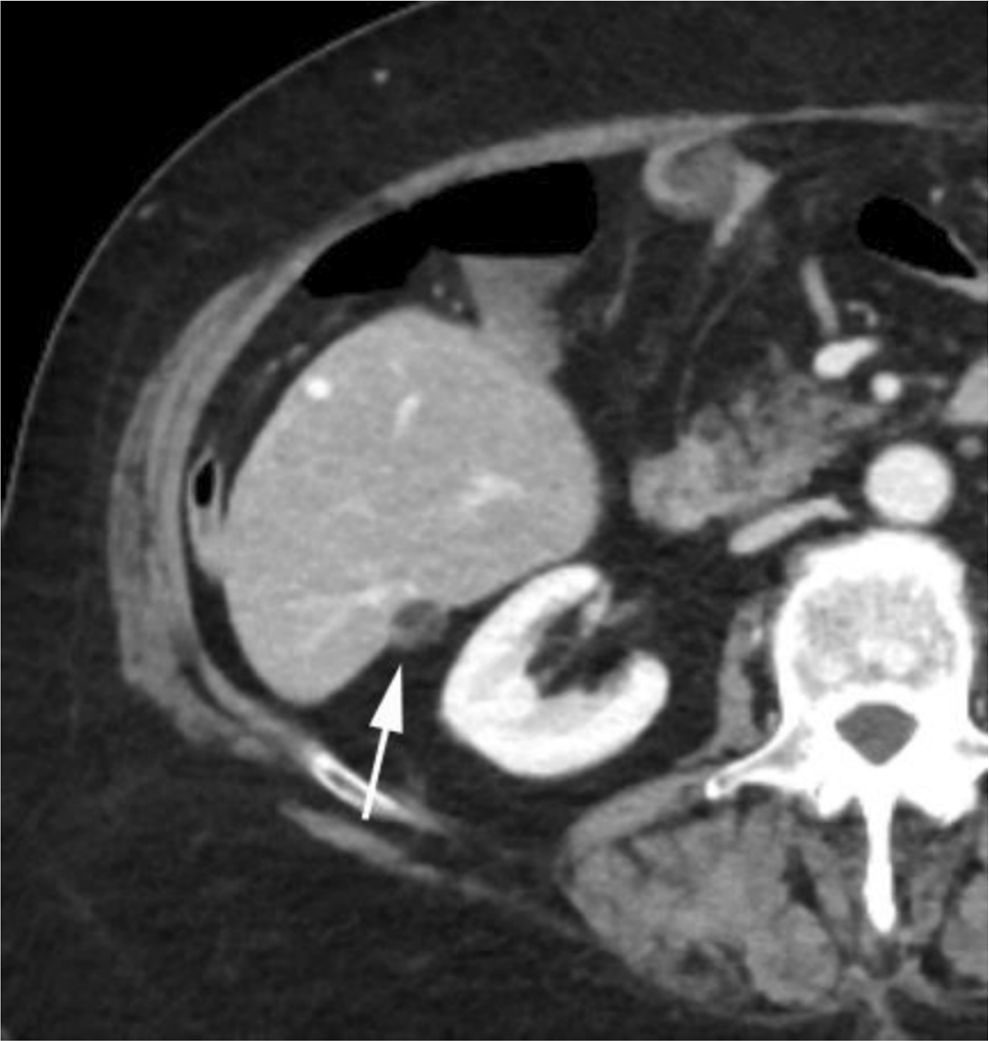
Portal venous phase CT showing a small fatty capsular implant (*arrow*) at the deep aspect of the liver within Morrison’s pouch in keeping with a pseudolipoma of Glisson capsule. It indents the underlying hepatic parenchyma
